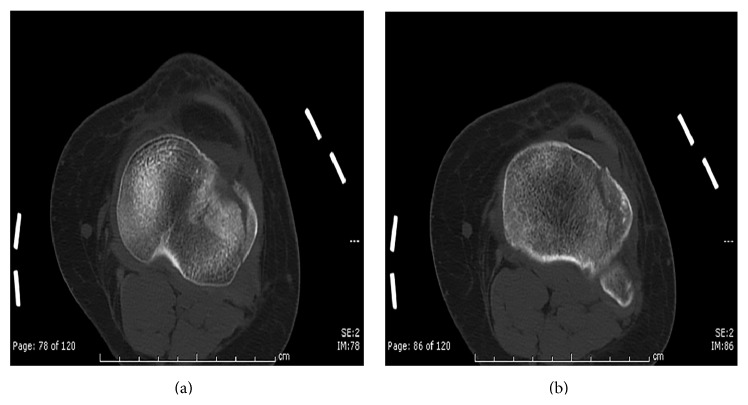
Initial CT scans of left tibia showing fracture of lateral tibial condyle.
